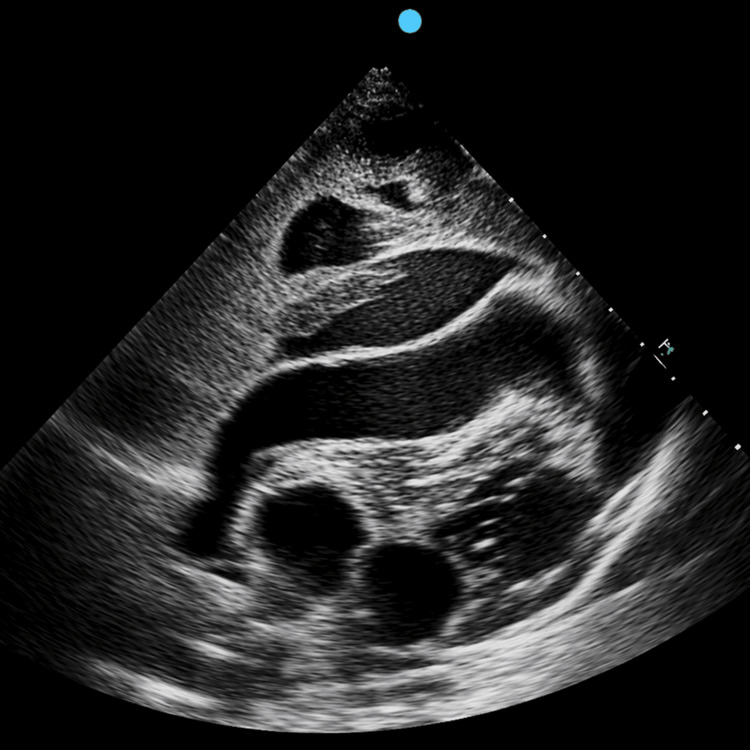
Initial POCUS showing pericardial effusion TTE demonstrated a large circumferential pericardial effusion with right ventricular diastolic collapse, consistent with cardiac tamponade. An additional atypical fluid collection was noted adjacent to the pericardium. POCUS: point-of-care ultrasound; TTE: transthoracic echocardiography
